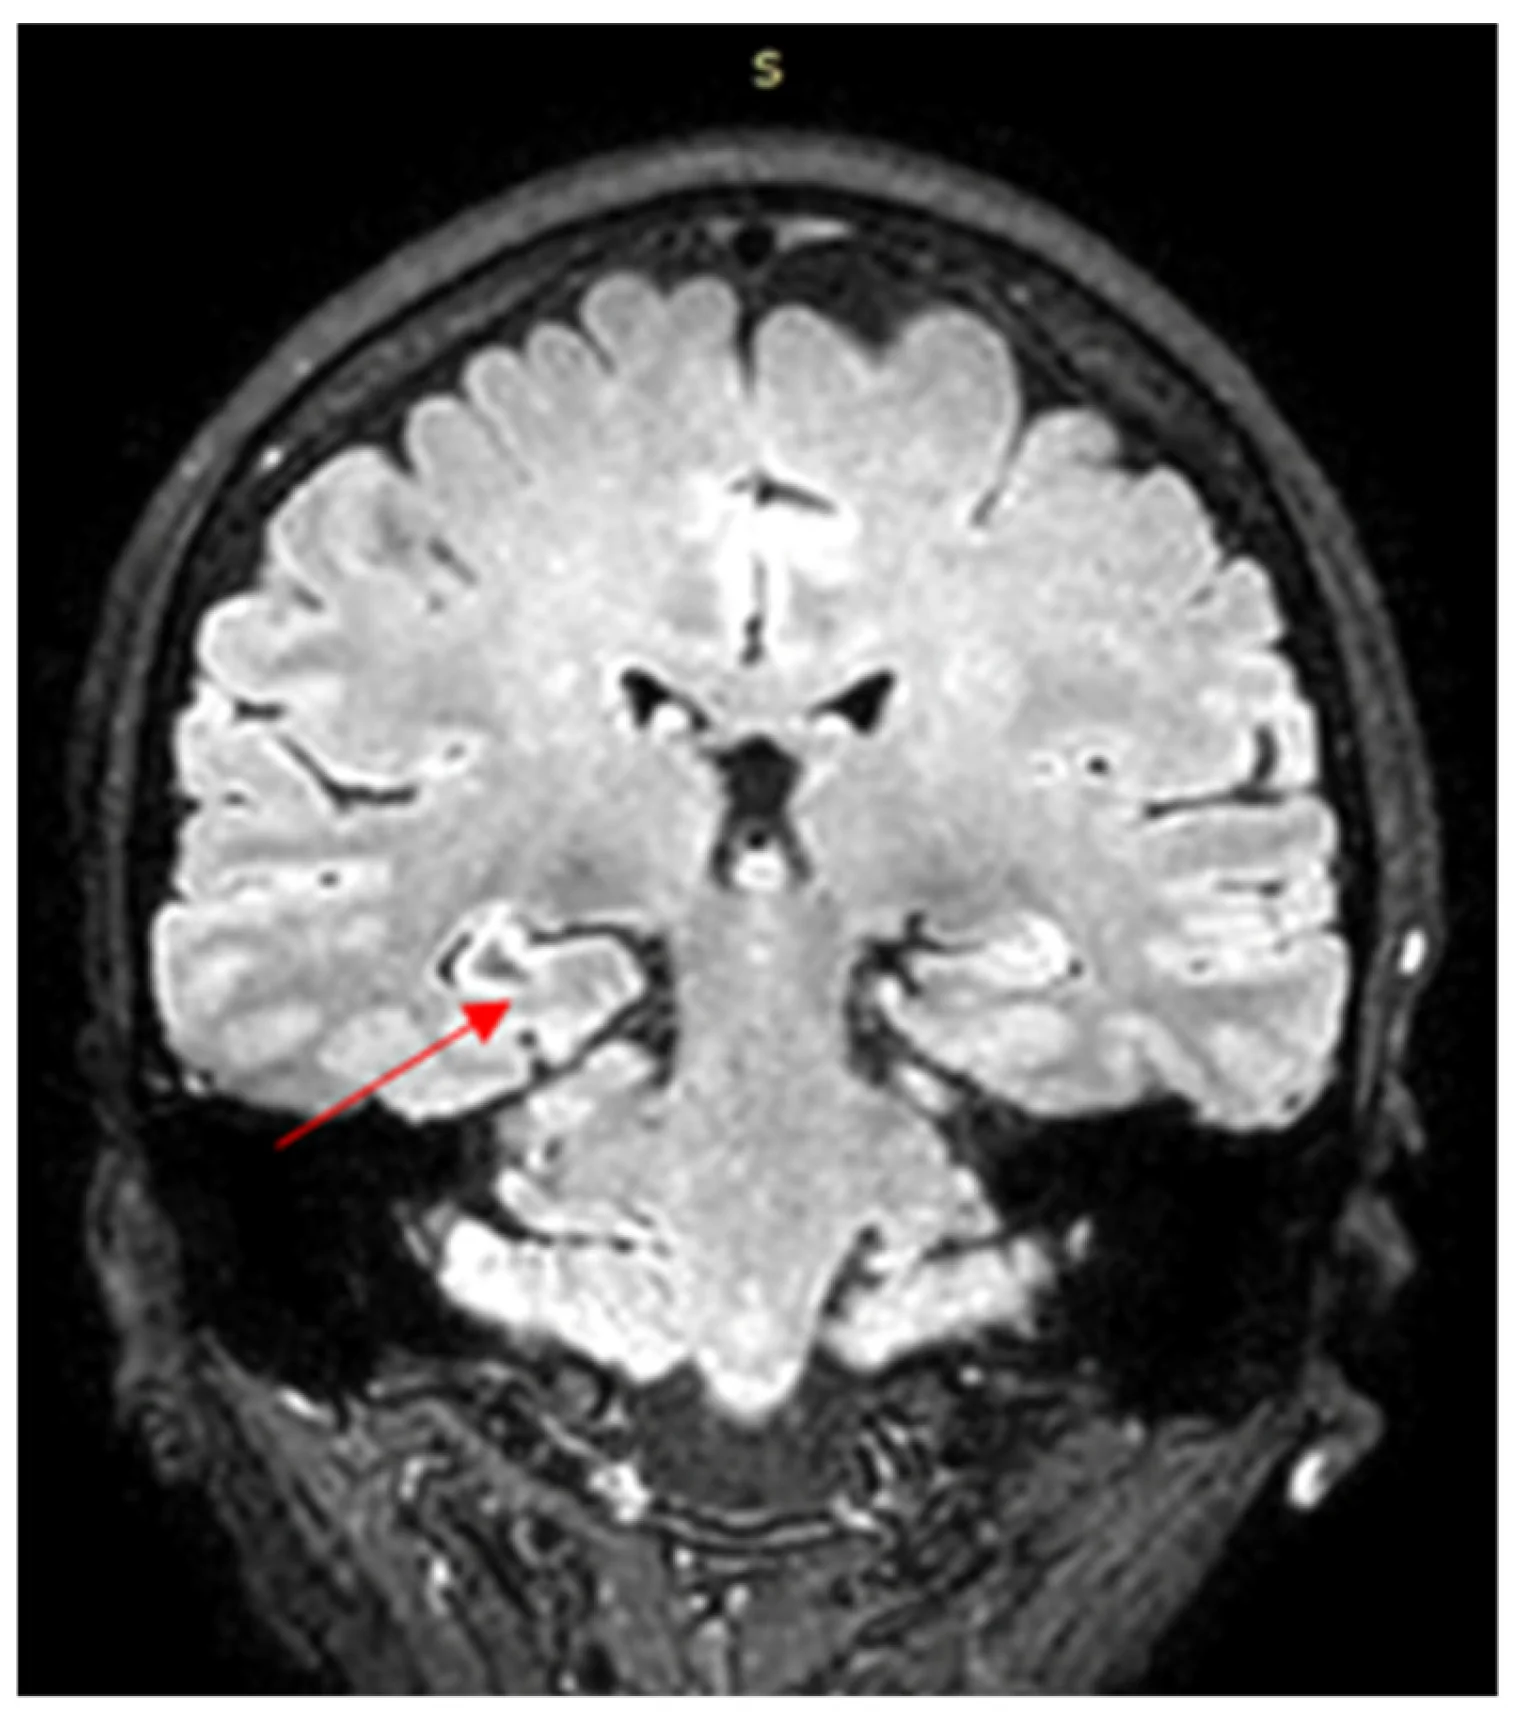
Whole-brain coronal MRI demonstrating the right hippocampal lesion (arrow). The image highlights the location and morphology of the lesion within the medial temporal lobe.
